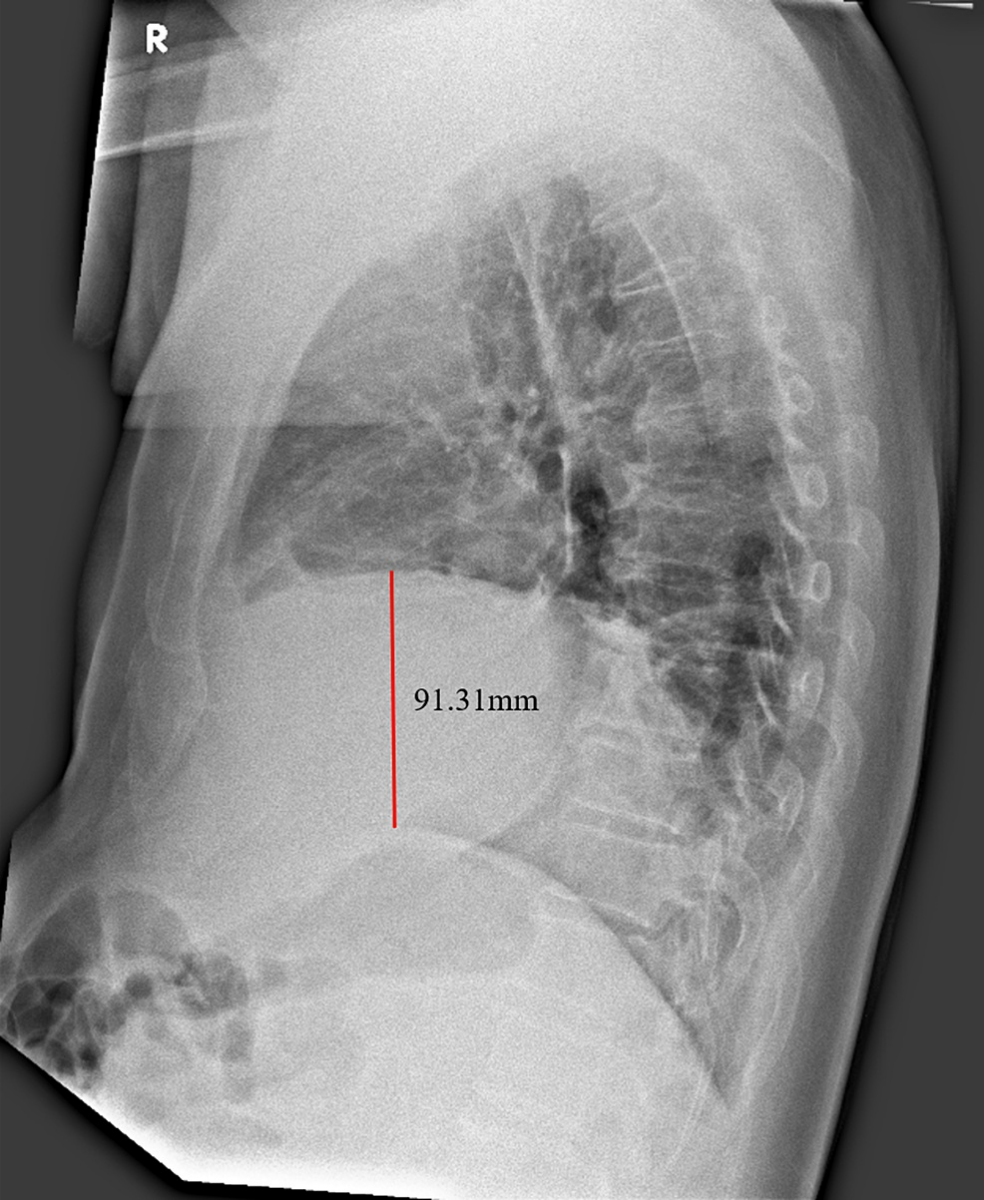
Erect right lateral CXR of Case 1 taken two days after admission. The right hemidiaphragm is markedly higher than the left. A small right pleural effusion is also present. CXR - chest X-ray
